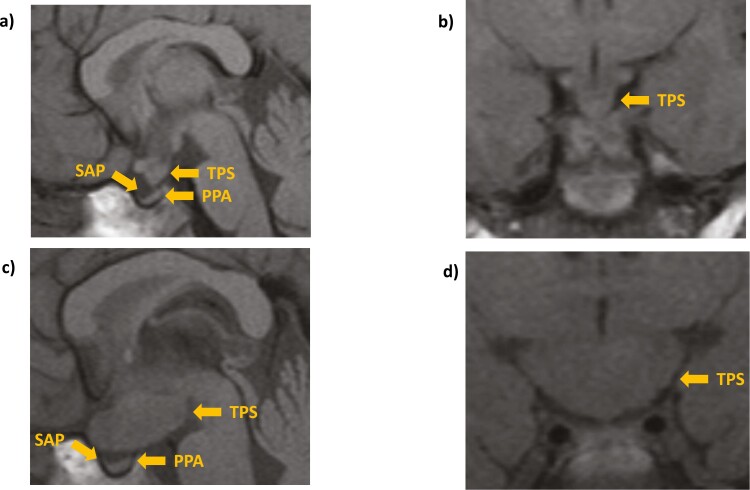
Brain magnetic resonance imaging—case 2. (A and B) Images taken at the age of 3.15 showing thickened pituitary stalk, absent posterior pituitary, and small anterior pituitary on (A) sagittal and (B) coronal views. (C and D) Images acquired at the age of 4.25 years showing marked enlargement of the suprasellar lesion on (C) sagittal and (D) coronal views. PPA, posterior pituitary absence; SAP, small anterior pituitary; TPS, thickened pituitary stalk.
